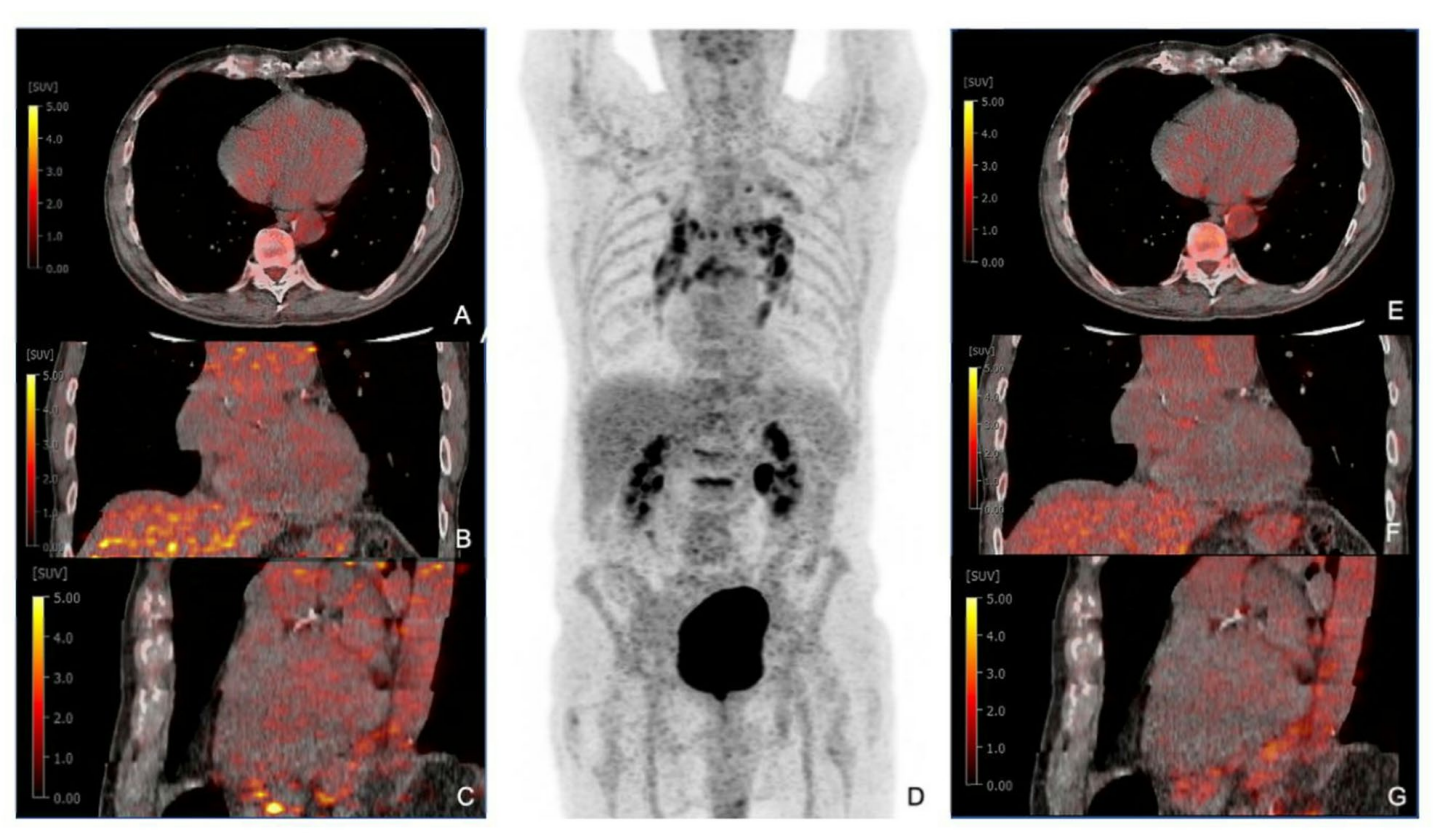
[^18^F]F-FDG PET/CT in patient 71 with suspected cardiac involvement in proven extraCS. A-C transaxial, coronar and sagittal PET/CT fusion after 60 min of FDG uptake time. D maximum intensity projection. E-G transaxial, coronar and sagittal PET/CT fusion after 90 min of FDG uptake time. No FDG uptake is detected. Extracardiac involvement is shown by hypermetabolism of bihilary lymph nodes
